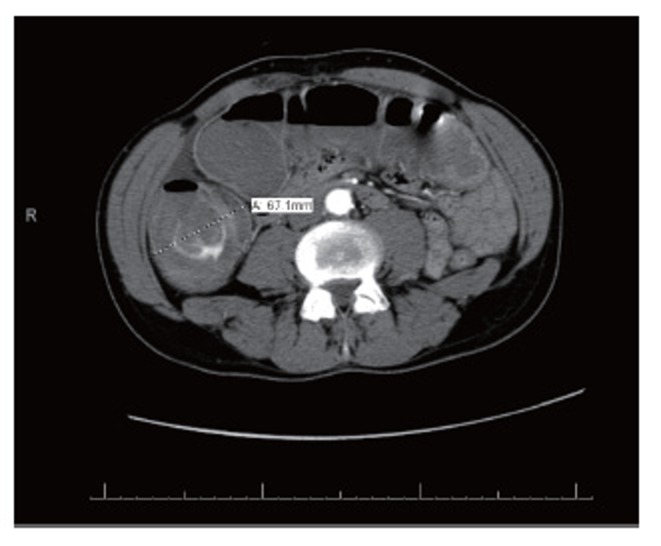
CT with oral and IV contrast on presentation. Cecum dilated to 6.7 cm.
